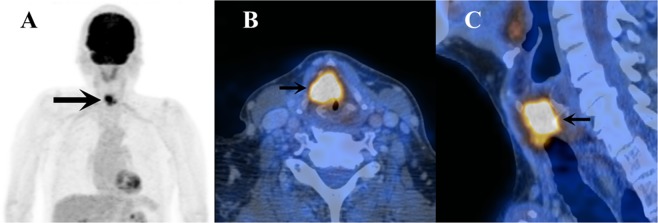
Example of a cT3 glottic carcinoma of the larynx in a 78-year-old patient. The tumor displays a SUV_max_ of 10.4, an MTV of 7 cm³, and a TLG of 45’830. (A) Frontal view of PET image. (B) Axial view of fused PET/CT image. (C) Sagittal fusion PET/CT image of the tumor.
